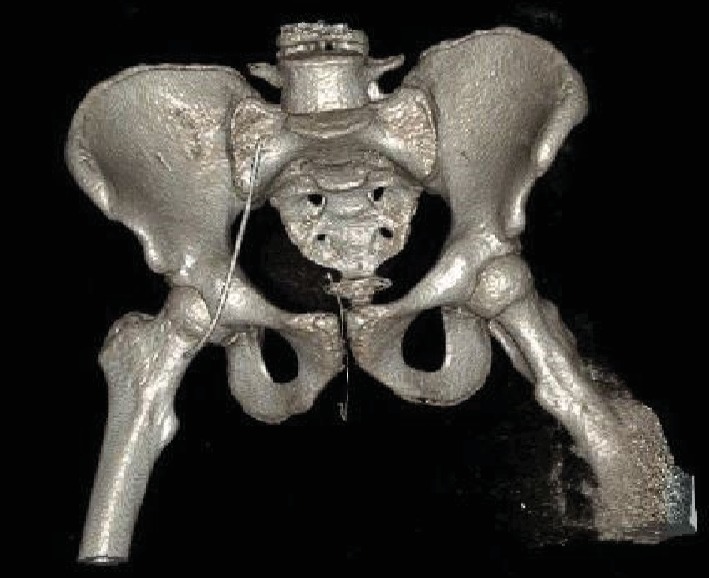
Postoperative 3D reconstruction from CT pelvis without contrast demonstrating reduced left femoral head without associated femoral fracture.
